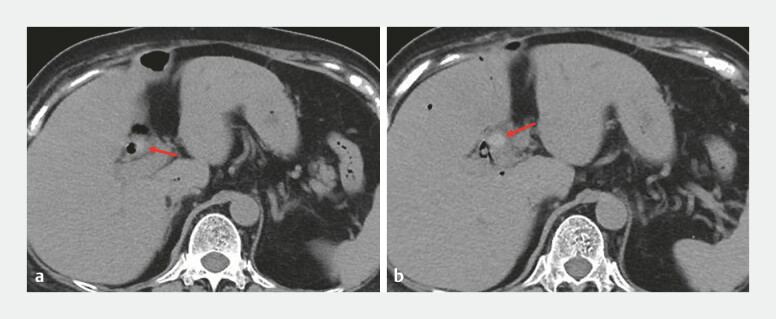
Computed tomography revealed stones (arrows) in the intrahepatic bile duct.
